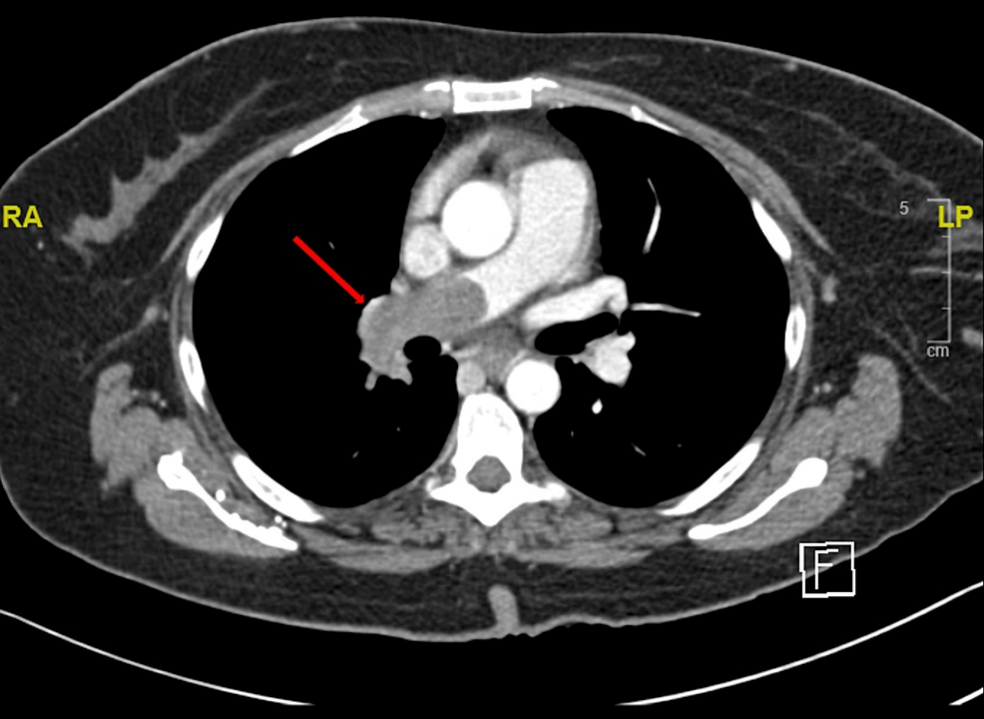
CT with contrast (axial view) of the chest showing pulmonary embolism (red arrow) of the right pulmonary artery.
